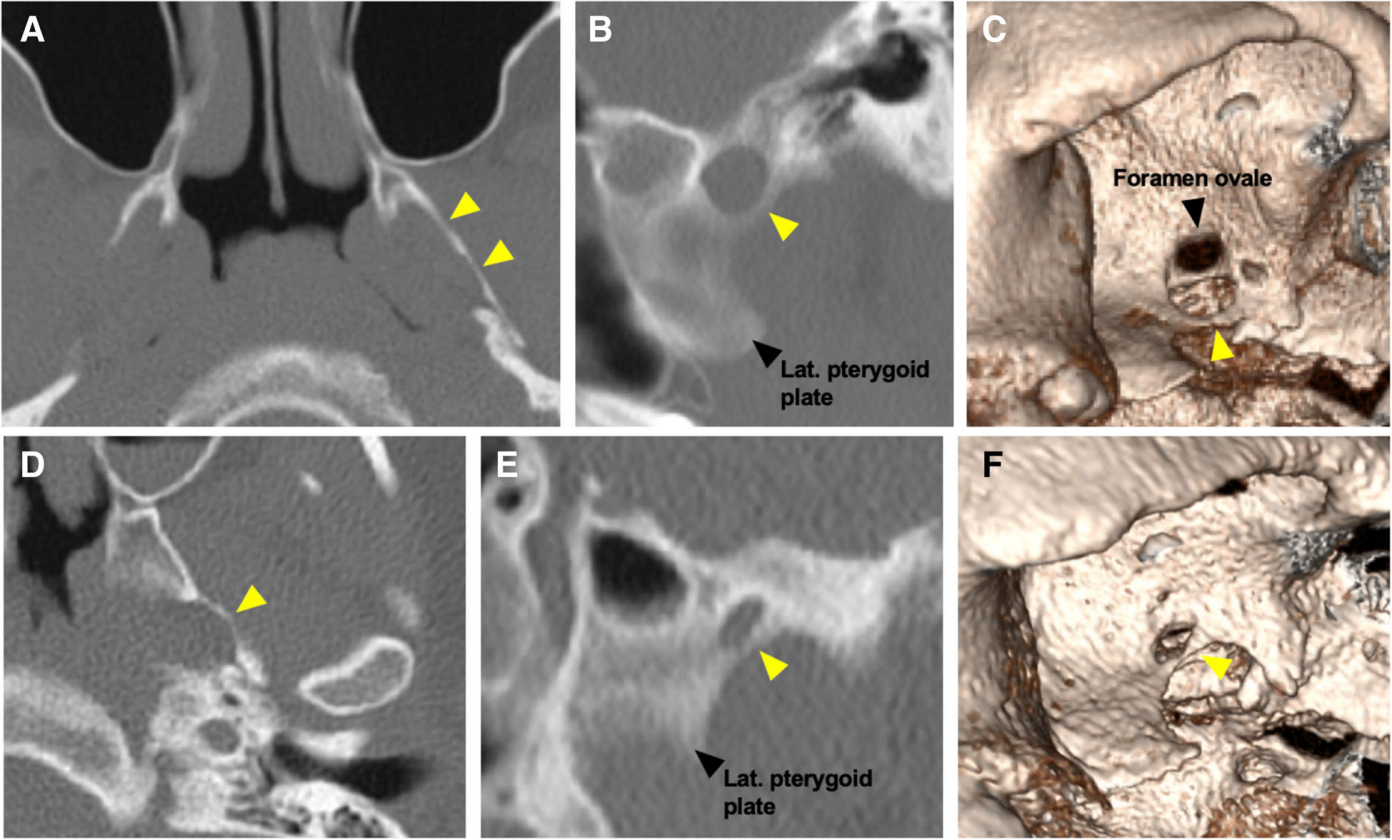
Mineralised pterygospinous and pterygoalar ligaments. Axial (a), oblique sagittal MIP (b), and 3D (c) volume CT reconstructions demonstrating a completely mineralised left pterygospinous ligament (yellow arrowheads). The foramen ovale is demonstrated on the 3D reconstruction. Axial (d), oblique sagittal MIP (e), and 3D (f) volume CT reconstructions demonstrating a completely mineralised left pterygoalar ligament (yellow arrowheads). The foramen ovale is obscured
